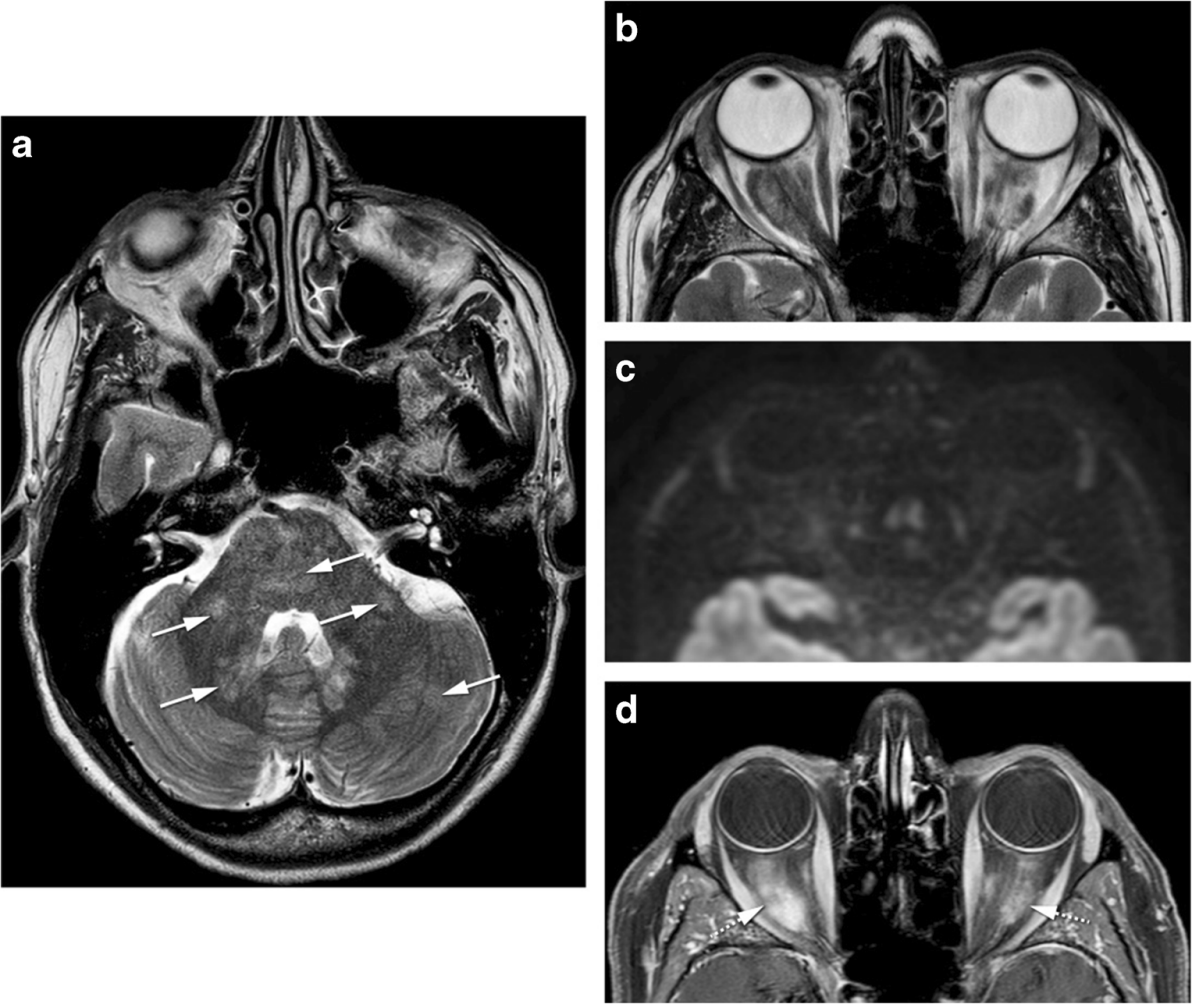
47-year-old female with Erdheim-Chester disease. Axial T2-WI (a, b), axial DWI (c) and enhanced axial T1-WI with fat signal suppression (d): bilateral intraconal masses surrounding the optic nerves (dashed arrows), slightly heterogeneous on T2, no diffusion restriction, enhancing after contrast. Bilateral involvement of cerebellum, middle cerebellar peduncles, and pons (arrows)
